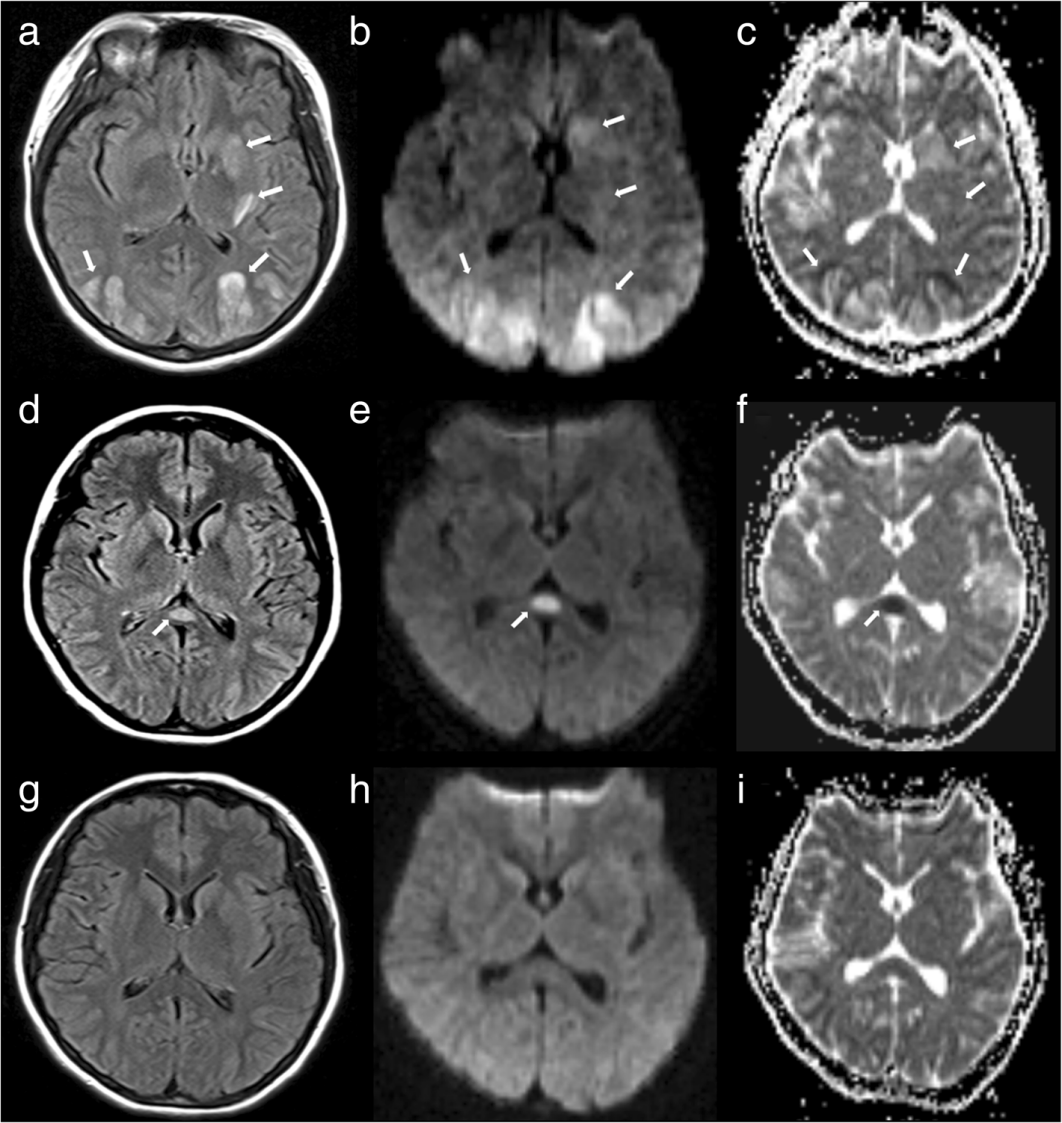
Brain magnetic resonance (MR) images for a 23-year-old woman with eclampsia who presented with sudden-onset headache, dizziness, and severe hypertension (blood pressure, 170/110 mmHg). a–c. Axial brain MR images obtained at the clinical onset of symptoms show vasogenic cerebral edema in the bilateral parietooccipital lobes, left basal ganglia, and corona radiata. Fluid-attenuated inversion recovery (FLAIR) imaging (a), diffusion-weighted imaging (DWI; b = 1000; b), and apparent diffusion coefficient (ADC) mapping (c) show mild hyperintensity in the lesions (arrows), which is a typical finding in posterior reversible encephalopathy syndrome (PRES). d–f. Follow-up MR images obtained 10 days after the clinical onset of symptoms (i.e., 1 day after withdrawal of mannitol show that the originally observed hyperintense lesions have disappeared (vasogenic edema has disappeared). FLAIR imaging (d) shows an isolated hyperintense signal in the splenium, whereas DWI (e) and ADC mapping (f) show restricted diffusion in the splenium (arrows), which is a typical finding in reversible splenial lesion syndrome. g–i. Follow-up MR images obtained 29 days after the clinical onset of symptoms FLAIR imaging (g), DWI (h), and ADC mapping (i) show that the lesions in the splenium have disappeared
